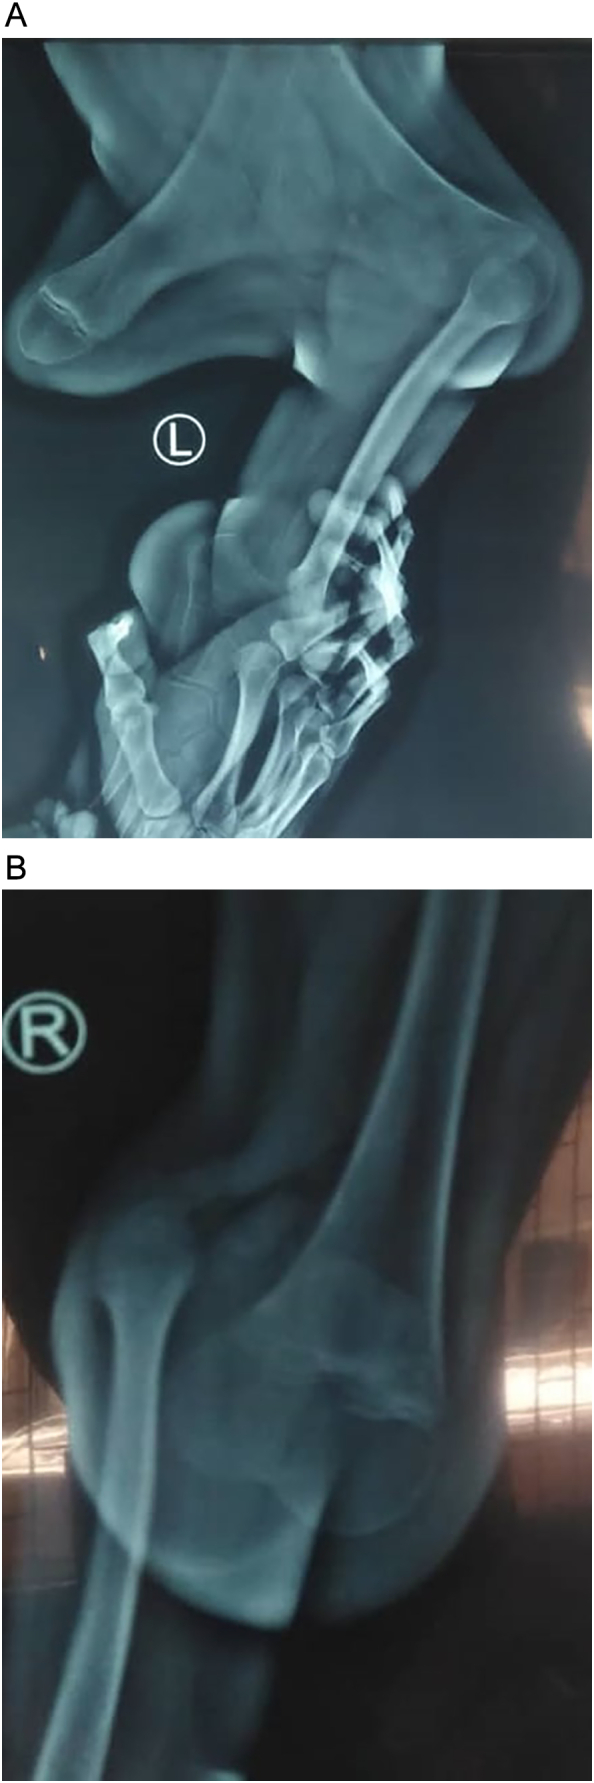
X-ray image of the left bifid femur and complete tibial hemimelia (A) and complete tibial hemimelia of the right limb (B).
